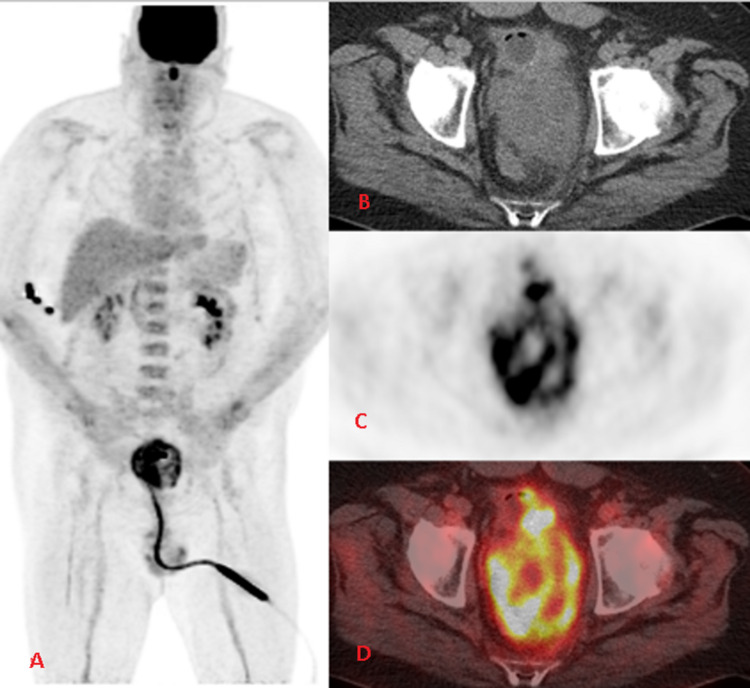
(A) FDG PET MIP showing heterogeneous FDG uptake in the prostate gland in addition to the physiologic distribution of the radiotracer; (B) Axial non-contrast CT showing heterogeneously enlarged prostate gland with interspersed areas of necrosis; (C) Axial FDG PET showing heterogeneous FDG uptake in the enlarged prostate gland; (D) Fused FDG PET CT showing heterogenous marked tracer uptake in the enlarged irregular prostate gland FDG: fluorodeoxyglucose; PET: positron emission tomography; MIP: maximum intensity projection
